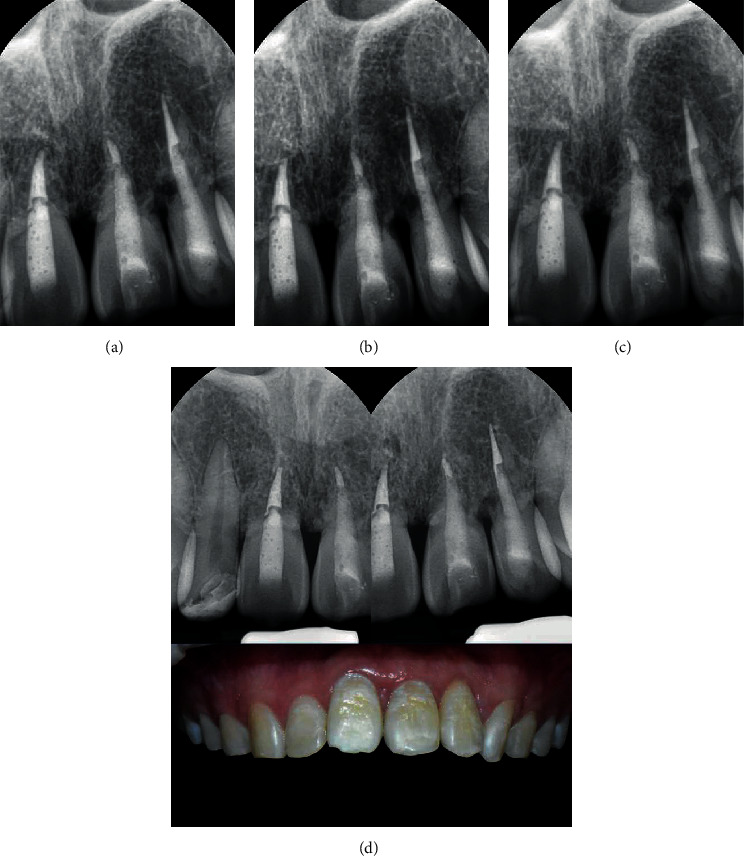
Periapical radiographs show gradual progression of root resorption. At the 9-year follow-up, clinical photograph of the avulsed teeth was also taken. ((a) 4 years: 10 m; (b) 5 years: 7 m; (c) 6 years: 2 m; (d) 9 years).
